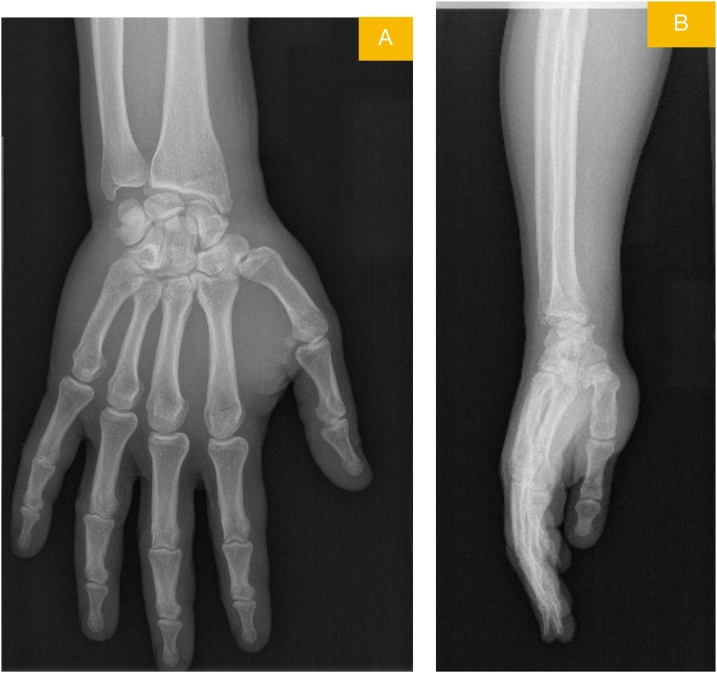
Posteroanterior (A) and lateral (B) view radiographs of wrist showing radial styloid. displaced and ulnar styloid minimally displaced fractures
